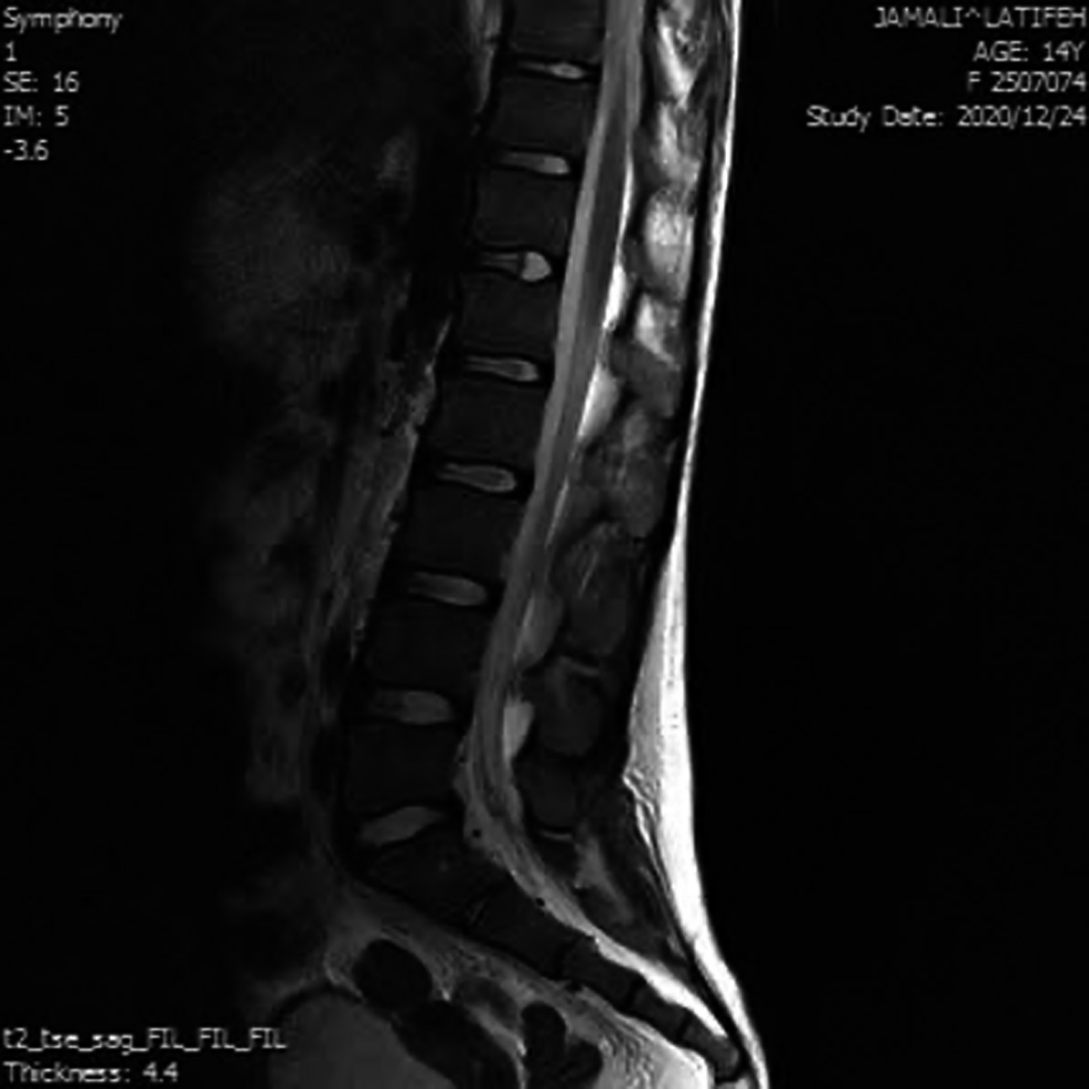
Thoracolumbosacral MRI revealing no evidence of spondylodiscitis adjacent to psoas collection
